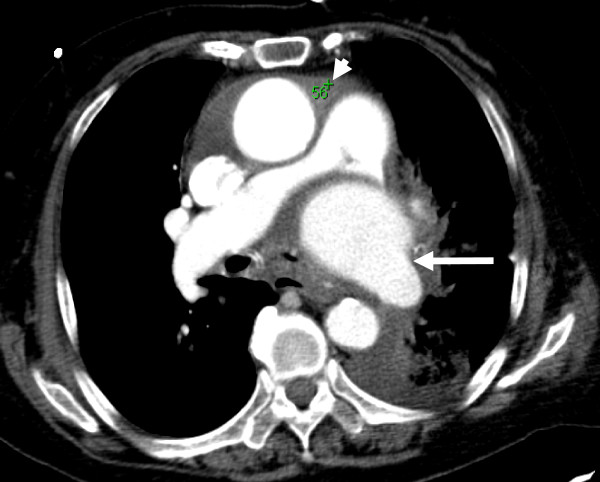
Cross section of chest CT: arrow (white) points the pseuoaneurysm, compression of pulmonary artery by pseudoaneuysm was noted. Pericardium effusion is identified in hyper-density (white arrow head) suggesting bloody component that may resulted from the pseudoaneurysm hemorrhage into pericardium space.
